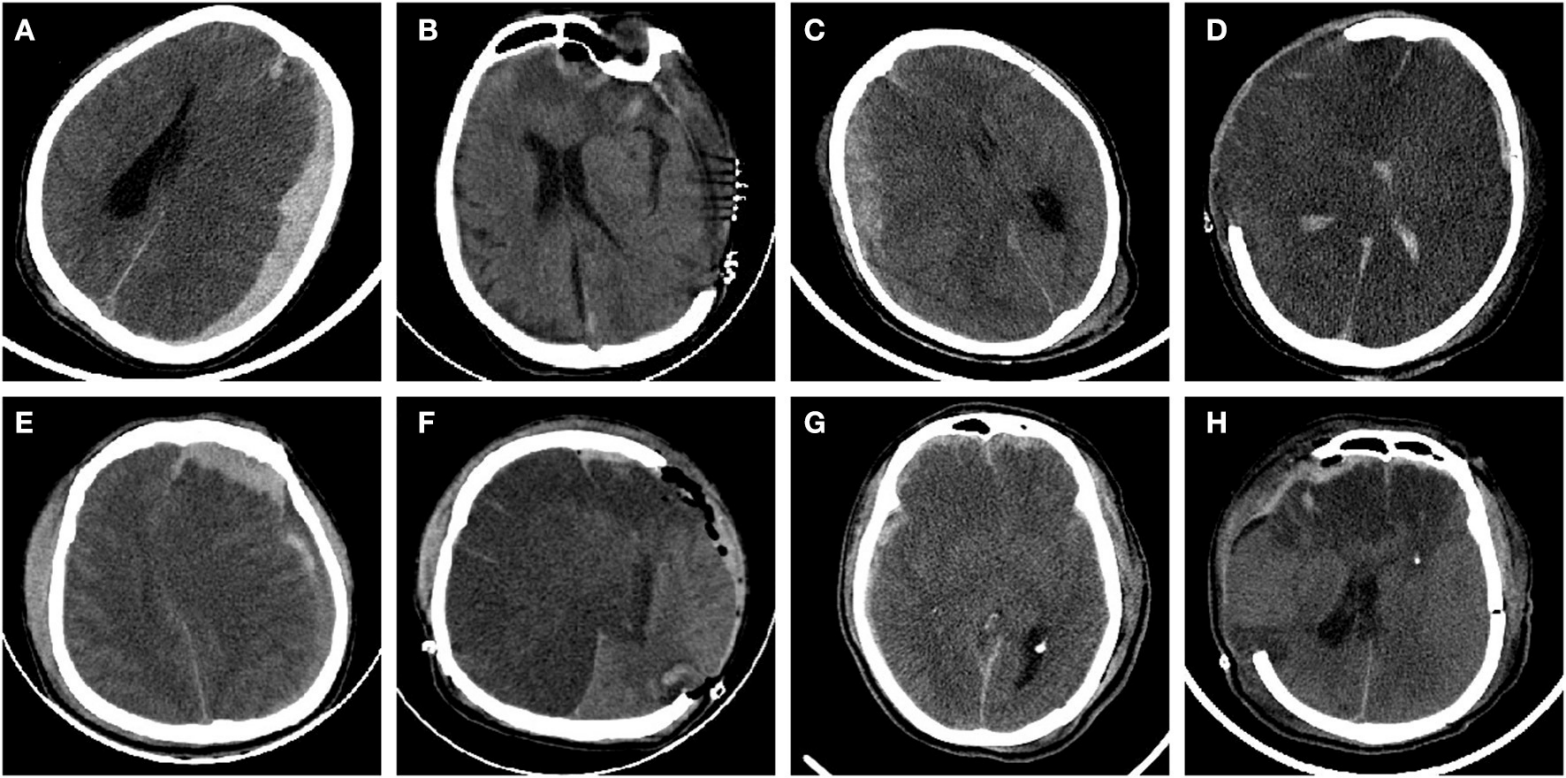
CT imaging of patients with secondary cerebral infarction after TBI cerebral hernia. (A) Left frontotemporoparietal hematoma after TBI. (B) Hematoma evacuation and decompressive craniectomy showing a left occipital low-density infarction area. (C) Right frontotemporal hematoma with SAH. (D) Decompressive craniectomy showing cerebral hemispheric infarction. (E) Left frontotemporoparietal epidural hematoma with arachnoid hemorrhage. (F) Hematoma evacuation and decompressive craniectomy showed extensive infarction of the right cerebral hemisphere. (G) Frontal lobe laceration, right frontotemporal hematoma, and arachnoid hemorrhage. (H) Postoperative frontal lobe infarction.
